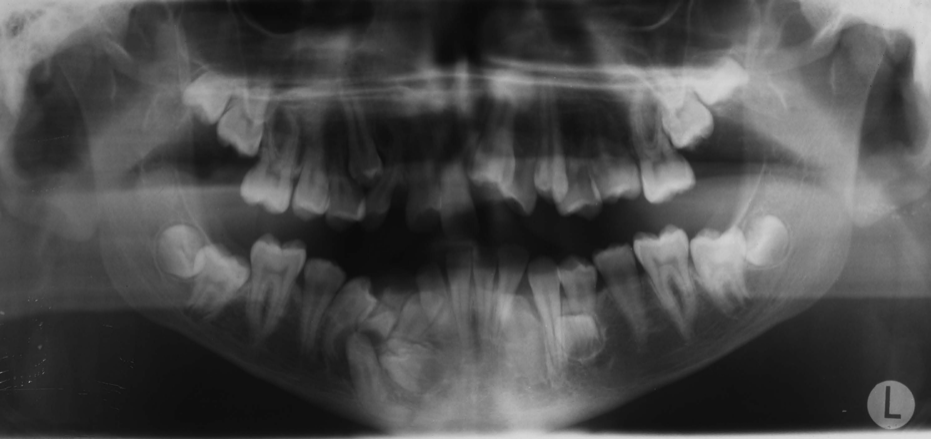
Orthopantomography at 13 years 9 months of age.
